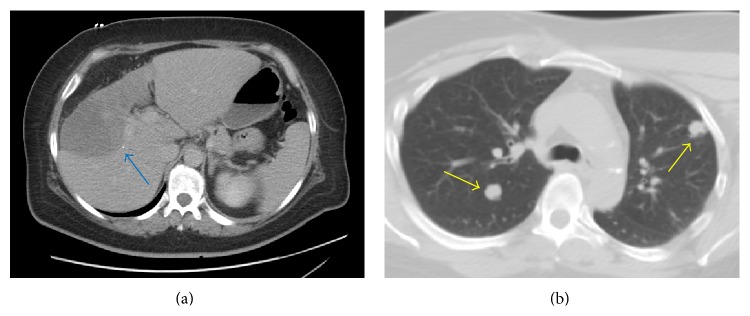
(a) Computed tomography (CT) of the abdomen showing a large hepatic mass, measuring 12 cm × 5.5 cm and designated by the blue arrow. (b) CT of the chest showing metastatic nodules, designated by yellow arrows.
